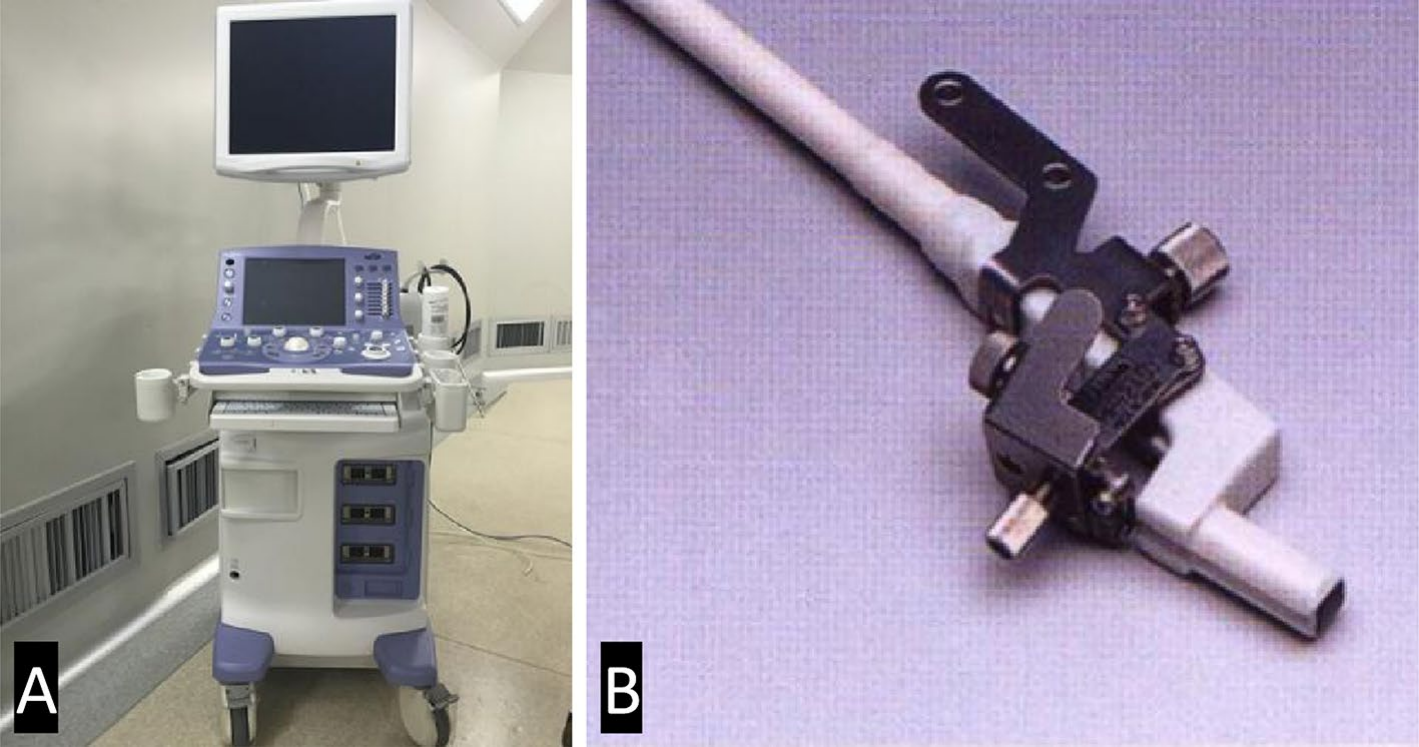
Intraoperative ultrasound equipment (A) and intraoperative electronic phased array probe for neurosurgical puncture (B).
